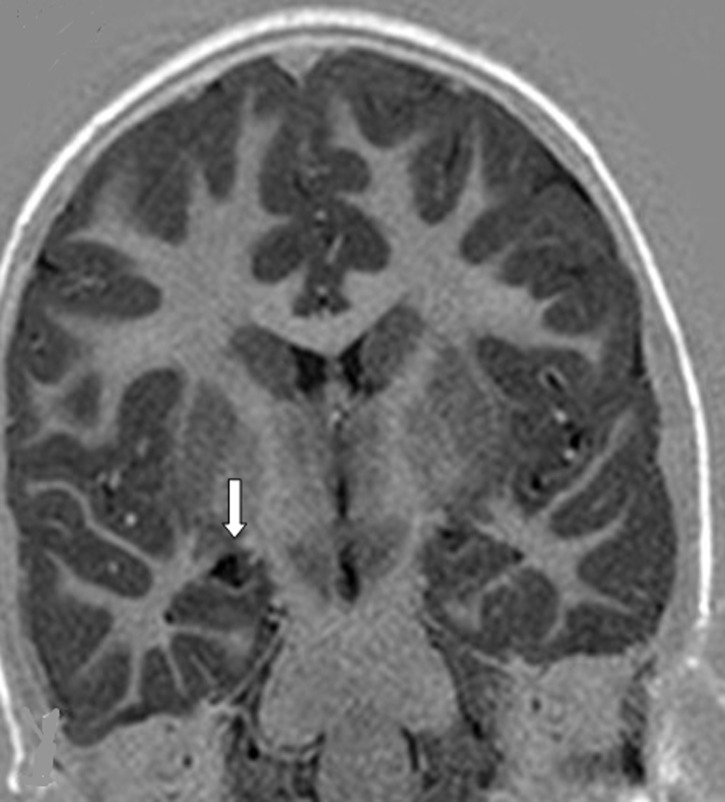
coronal MRI T1 inversion recovery showing a round cystic lesion of the right choroidal fissure compressing the hippocampus, hyposignal T1 (arrow)
